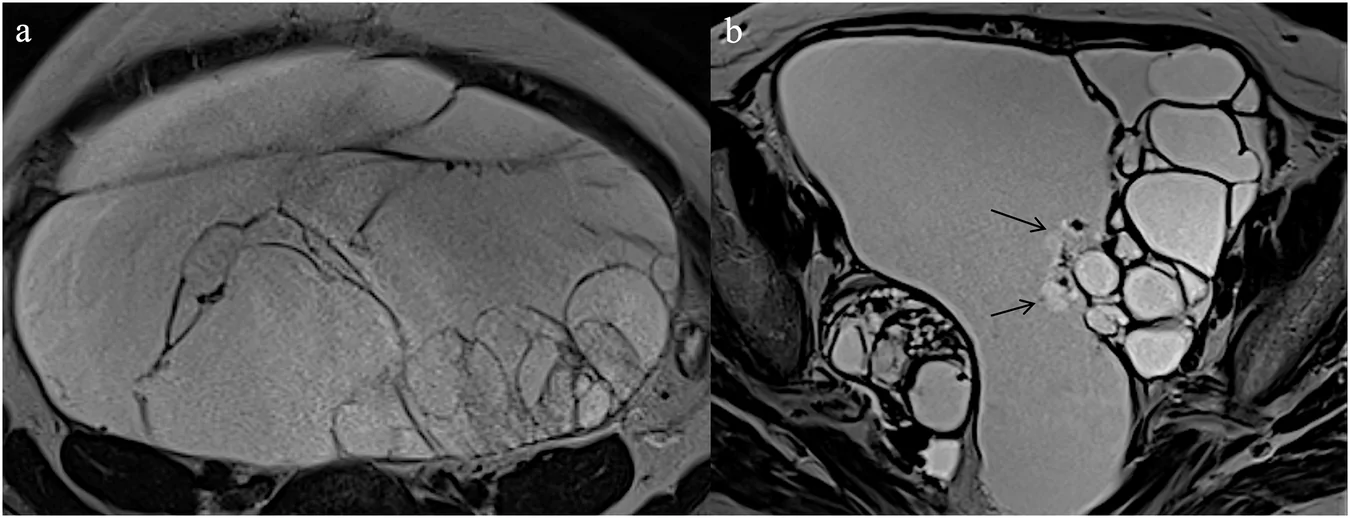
Mucinous borderline tumor and cystadenofibroma. a, b Axial T2-weighted sequences. a A typical mucinous borderline tumor and b a typical cystadenofibroma. Both appear as large multicystic tumors. Cystadenofibroma specifically shows low-T2 fibrous tissue, thickened regular fibrous septa, and papillary projections (black arrows), with bilateral involvement
